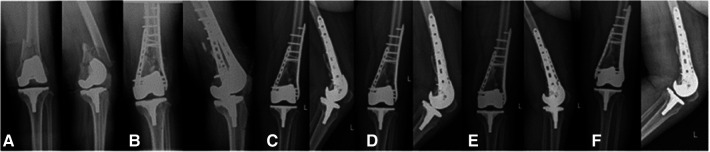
Anteroposterior and lateral radiographs of the left knee: preoperative x-ray image (A), postoperative x-ray images (B), radiograph 7 months after surgery (C), radiograph 9 months after surgery (D), radiograph 21 months after surgery (E), radiograph 34 months after surgery (F).
